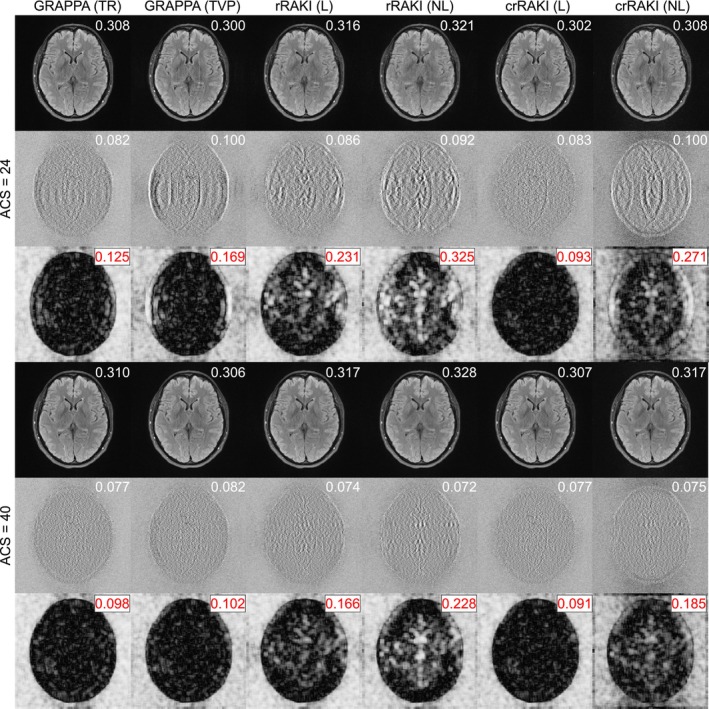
Comparison of the reconstruction for FastMRI FLAIR dataset id 200_6002425 slice index 0 for $R_{y}$ = 4 and two ACS sizes (24 and 40, $R_{eff}$ = 3.27 and 2.91, respectively). For each ACS set, the first row if the reconstructed magnitude image, the second row is the residual, the third row is the COBRA map. The numbers on the top‐right corners are respectively the Blur metrics, the mean RMSE, the COBRAI taken over a brain mask. GRAPPA with Tikhonov Regularization (TR) and Train‐Validation Partition (TVP), as well as rRAKI linear (L) and nonlinear (NL) perform well only when ACS size is large enough. rRAKI (NL) for $N_{y}^{acs}$ = 40 appears to be the one with lower NRMSE, but with significant structured residual artifacts. The crRAKI Linear method demonstrates superior capability in reconstructing high‐quality images with enhanced fidelity and accuracy.
